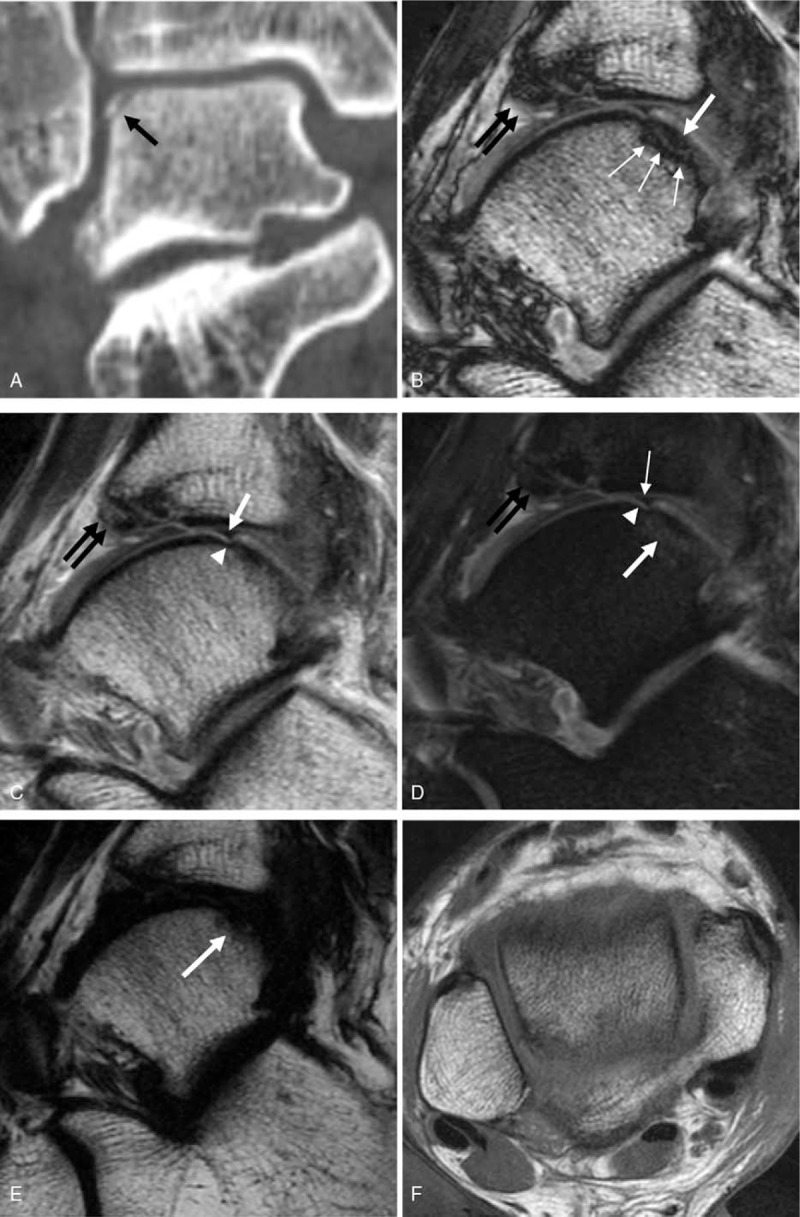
In lower leg CT (A), the chip fracture of the lateral talar dome is well depicted (arrow). There is no significant displacement of the fracture fragment. (B) Sagittal opposed-phase imaging from T2-weighted mDixon imaging demonstrates a wafer-shaped chip fracture at the corresponding area noted from the CT (white thick arrow). The fracture line is clearly delineated by a linear thick dark line caused by black boundary artifact (white thin arrows). (C) Sagittal in-phase imaging from T2-weighted mDixon technique shows a focal wedge-shaped cartilage defect at the talar dome (white arrow). There is subtle cortical irregularity at subjacent cortex (arrowhead). The fracture fragment is not delineated. (D) Sagittal water-only sequence from T2-weighted mDixon imaging shows a fuzzy linear bone marrow edema (white thick arrow). The cartilage defect (white thin arrow) and cortical irregularity (arrow head) are noted. (E) Sagittal fat-only imaging from T2-weighted mDixon imaging demonstrates a focal dark signal alteration at the subcortical area (arrow) which cannot be distinguished from the adjacent cortex or physiologic effusion. (F) In axial T1-weighted image, the fracture line is not clearly depicted. Note the displaced fracture fragment from the anterior tibial plafond is relatively clearly noted in B–D (black double arrows).
